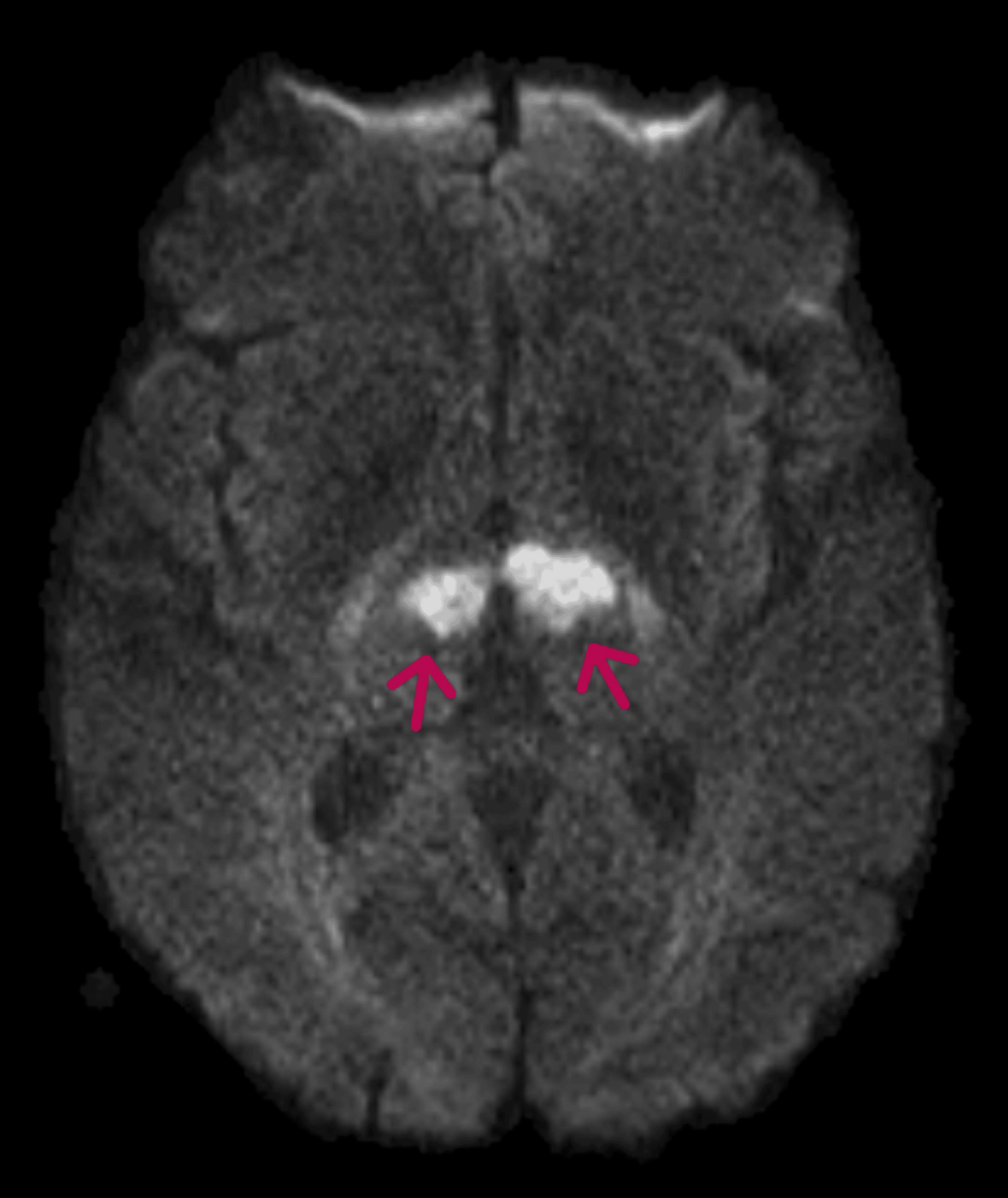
MRI brain (DWI sequence) showing diffusion hyperintensity in the bilateral thalami (arrows). DWI: Diffusion-weighted imaging
